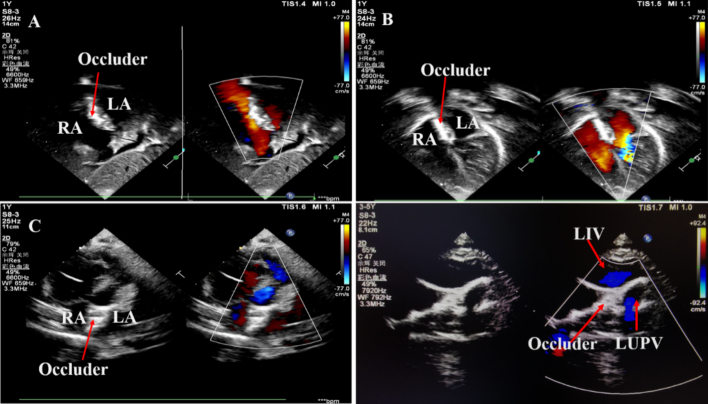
A–C: The occluder is visible in the atrial septum, with good shape and no residual shunt in the three views (subcostal two atrium chamber view, apical four-chamber view, parasternal short axis view) by 2D transthoracic echocardiography with color Doppler. D: The occlusion device is in the vertical vein and there is no obstruction of subclavian artery flow in the suprasternal fossa view by 2D transthoracic echocardiography. Notes: LA: left atrium; RA: right atrium; ASD: atrial septal defect. LIV: left innominate vein; LUPV: left upper pulmonary vein.
